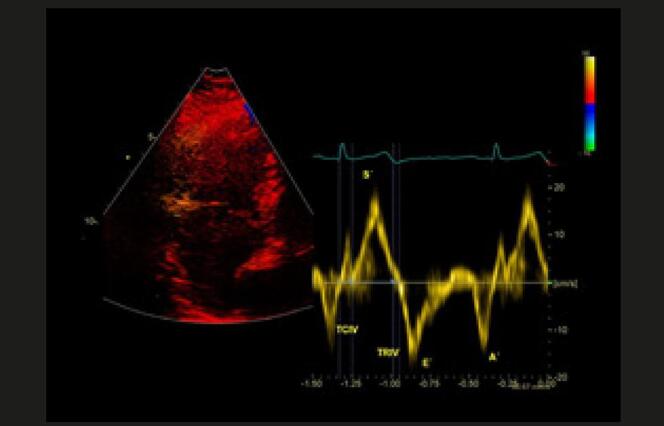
Velocidade de pico sistólico do anel tricúspide avaliado por meio do uso de *Doppler* tecidual pulsado (cm/s), através da janela apical e corte de 4 câmaras focado no VD. Importante manter segmento basal e anel alinhados com o *Doppler* para evitar subestimativa dessa medida. VN = 14,1 ± 2,3cm/s (>9,5cm/s)
